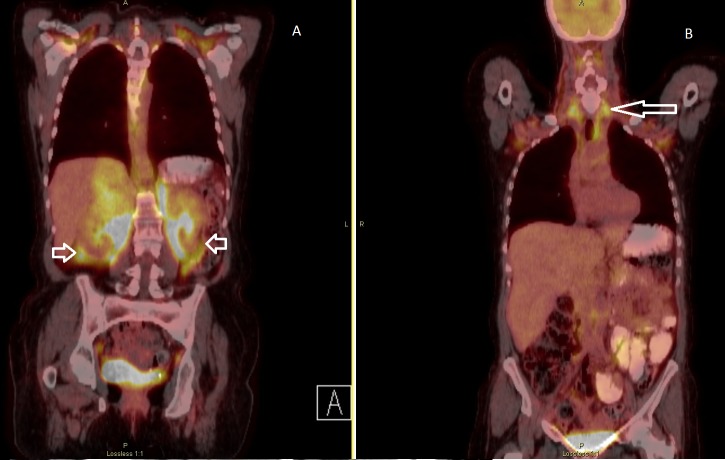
A positive emission tomography (PET) scan combined with computed tomography (CT) (PET/CT) (March 2016) reveals heterogenous moderate fluorodeoxyglucose (FDG) uptake in the large right adrenal gland lesion, with areas of central photopenia, which likely correspond to central necrosis. The extension of the abnormal FDG uptake to involve the para-aortic and aortocaval regions is noted (short arrows, Figure 2A). Also noted is the usual configuration of extensive brown fat uptake of FDG in the neck and thorax (long arrow, Figure 2B).
